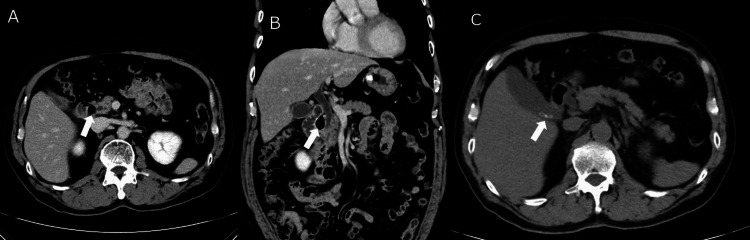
Contrast-enhanced abdominal CT on admission (A) Axial and (B) coronal reformatted images demonstrate an air-filled periampullary duodenal diverticulum (white arrows) abutting the ampulla and compressing the distal common bile duct. The upstream extrahepatic bile duct is dilated. No choledocholithiasis is identified in the bile duct. (C) The axial image shows multiple 3-mm gallstones (white arrow) within the gallbladder.
